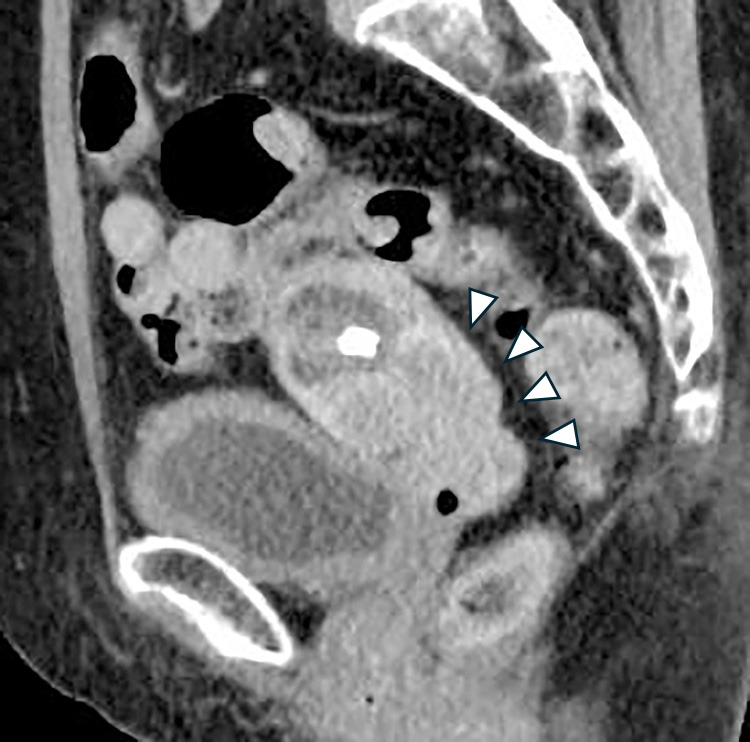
Non-contrast sagittal CT of the pelvic region showing a cervical mass (arrowhead).
